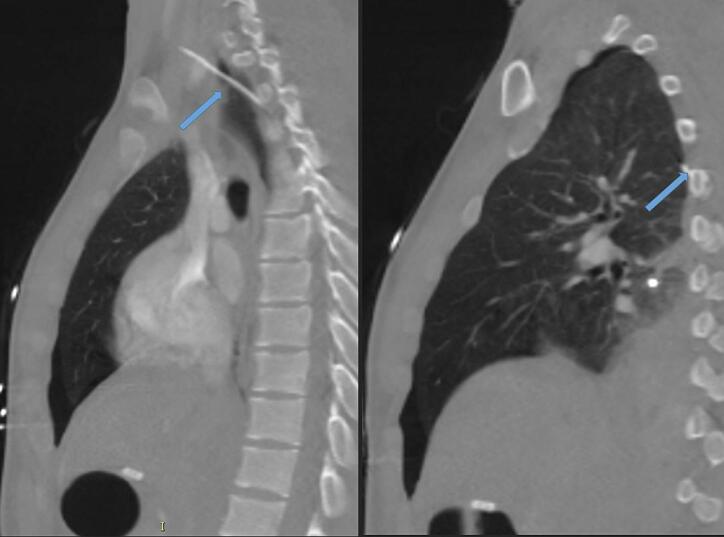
(a) Sagittal view of CT scan revealing puncture through the right subclavian artery (arrow). (b) Sagittal view of CT scan reveals formation of pneumothorax from gun-shot wound.
